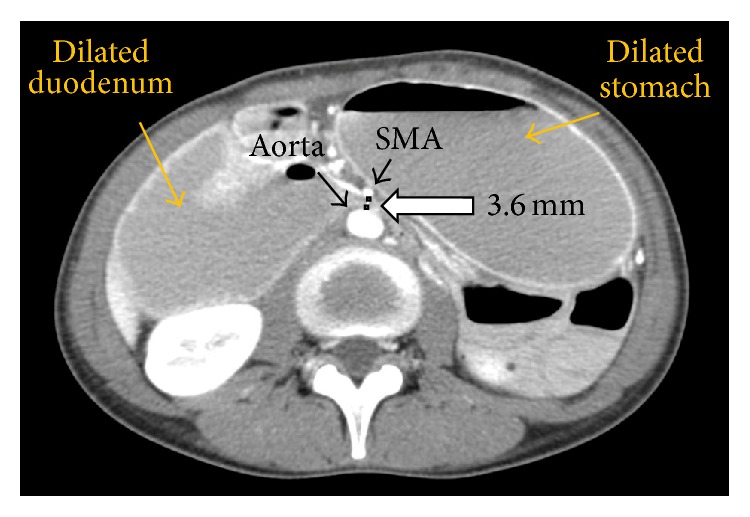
Contrast CT of the abdomen showing the distance between aorta and SMA (white arrow, marked as 3.6 mm). Also seen are a dilated stomach and duodenum and location of the SMA (black arrow).
